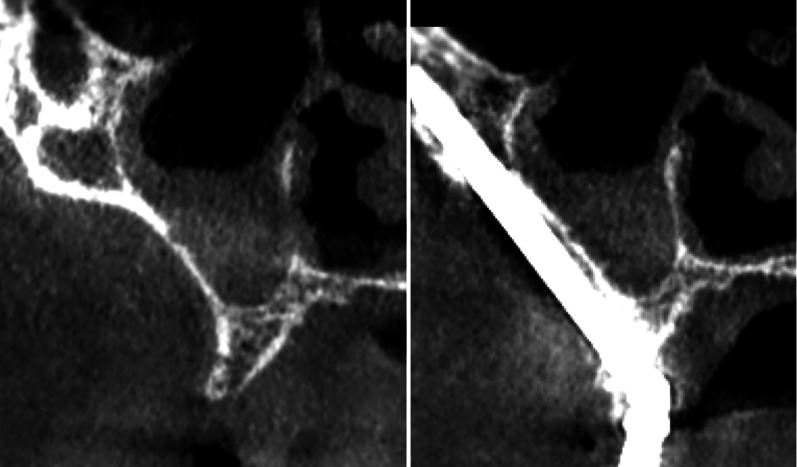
Preoperative CBCT images are compared to one-year postoperative images. Although there is postoperative sinus opacity, the Modified Lund–Mackay score [20] would be negative because the referred opacity existed before the surgery and did not increase afterward
